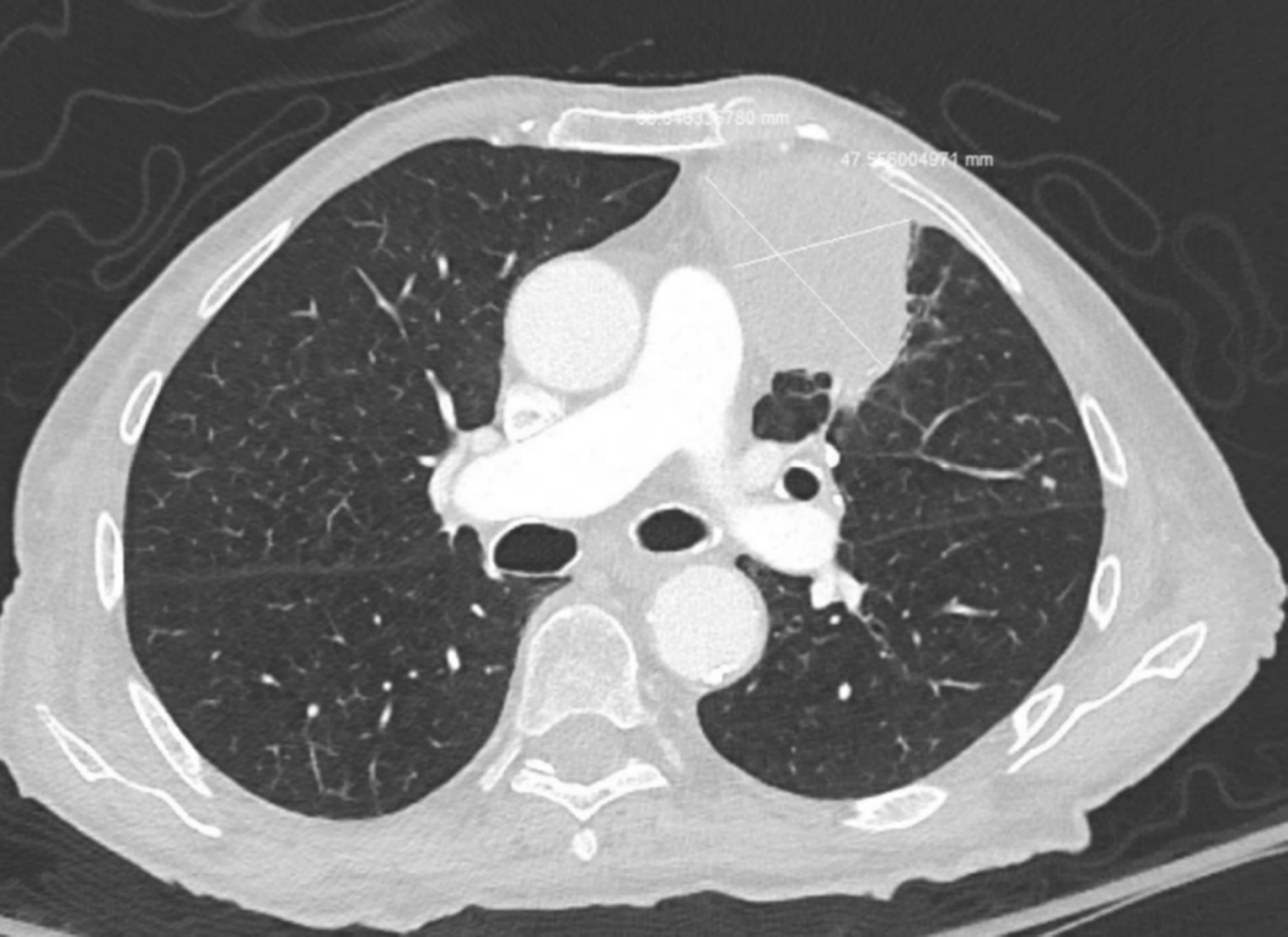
CT scan showing lingular mass abutting the mediastinal margin, mediastinal fat, and chest.
